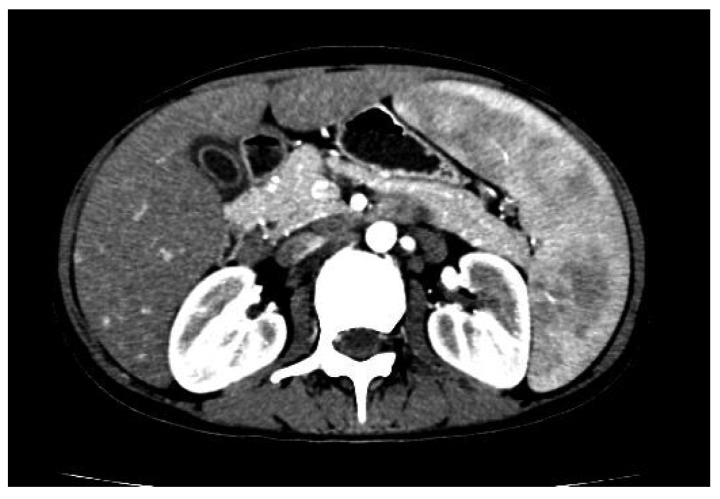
Abdominal computed tomography (CT) scan of the patient with Felty’s syndrome (FS).
